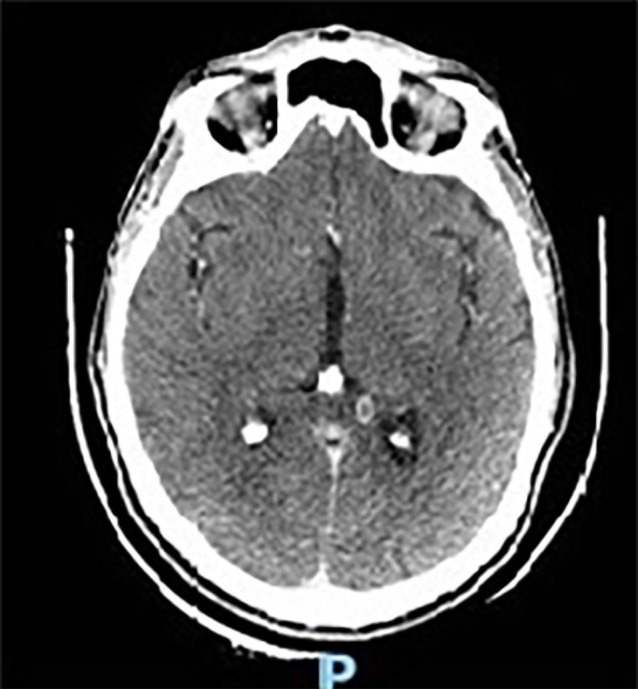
High-resolution computed tomography scan of the brain showing a
